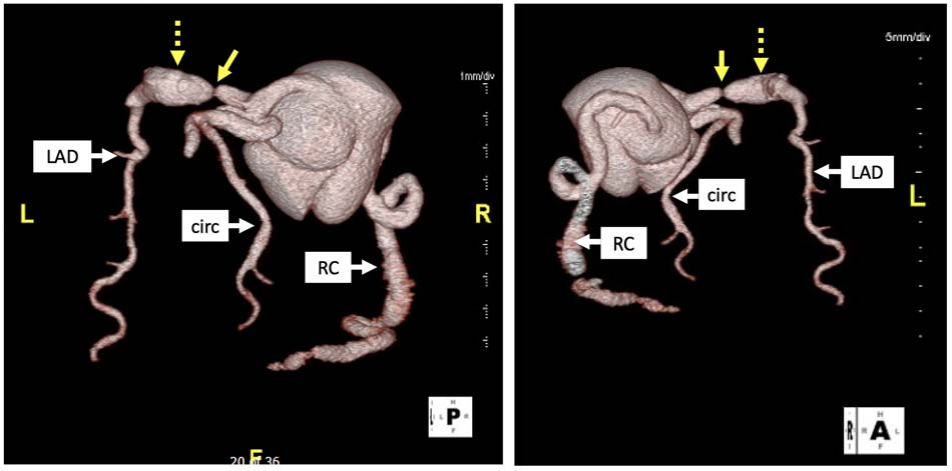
Computed tomography with 3D reconstruction. Solid yellow arrow points to tight stenosis in the left anterior descending and dotted yellow arrow points to post-stenotic aneurysm.
